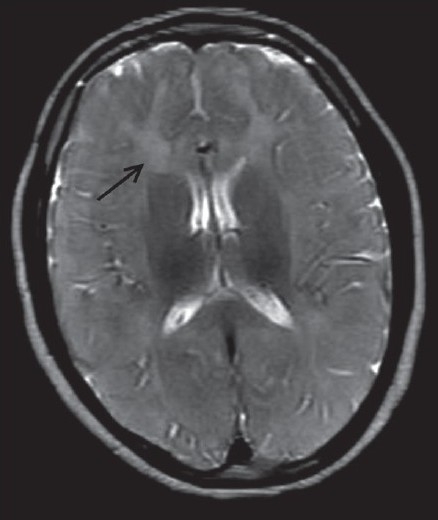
Magnetic resonance imaging T2W sequence in a 11-year-old child showing diffuse white matter hyperintensity (arrow) in the supratentorial white matter
